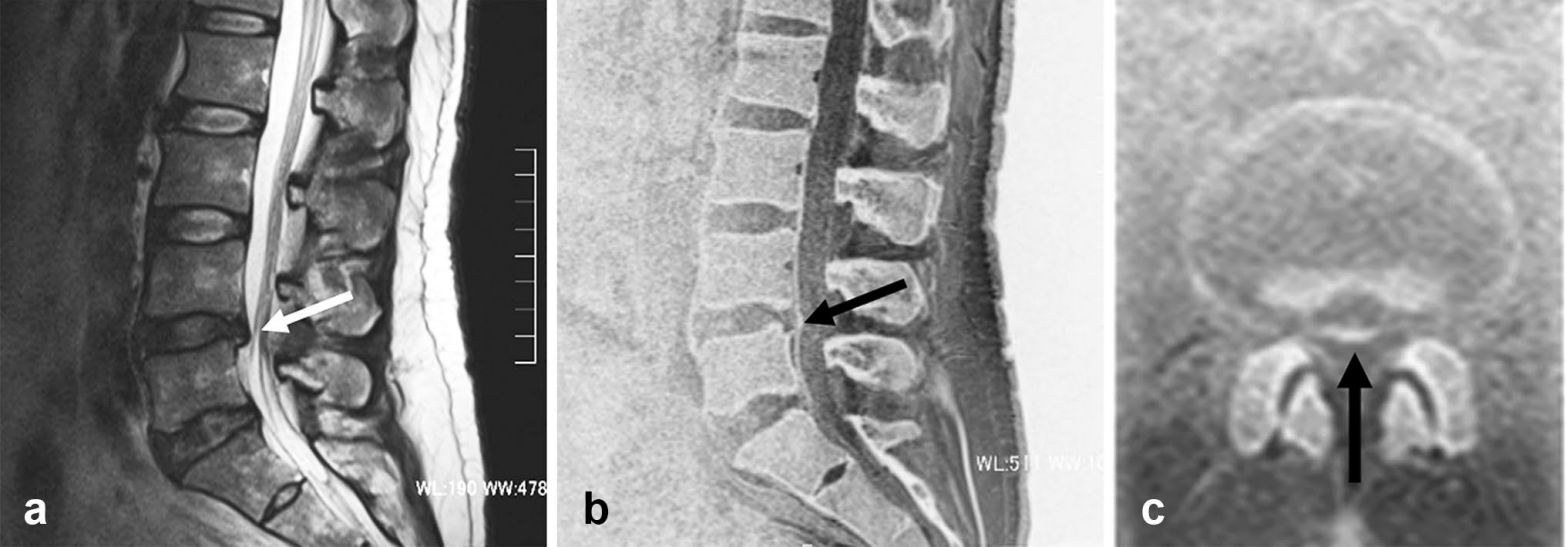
Lumbar disc herniation. Sagittal T2-weighted MR image a shows a herniated L4-5 disc in a 45-year-old male patient (arrow). Sagittal and axial MR bone images (b and c) show the herniated disc and preserved posterior longitudinal ligament (arrow)
